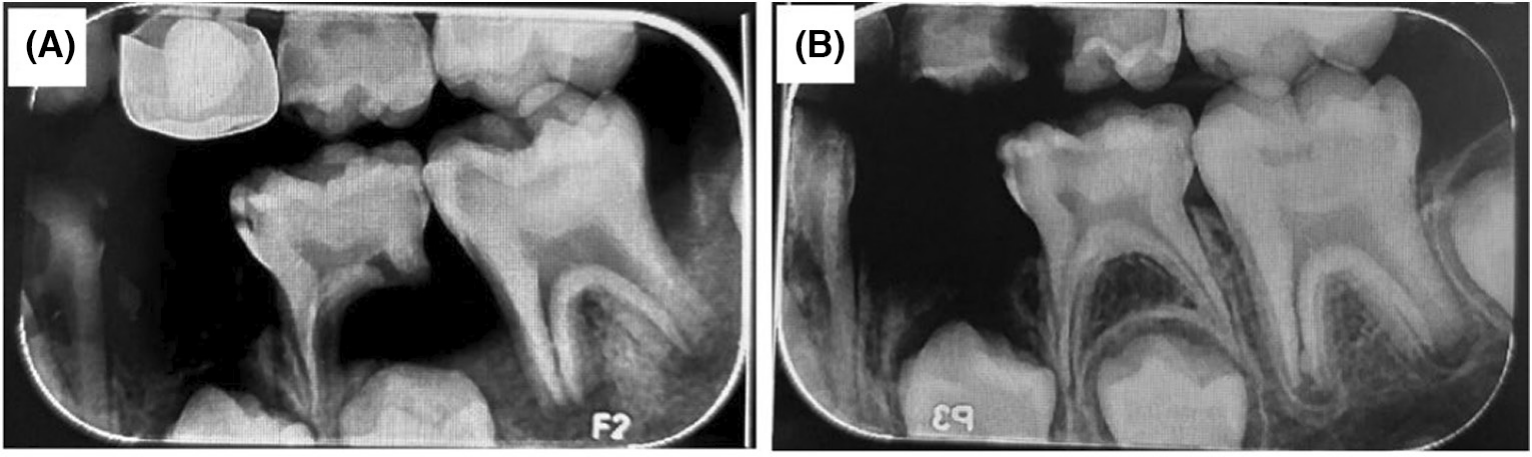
Pre‐operative radiograph, (A) Tooth #19 and (B) Tooth #30.
